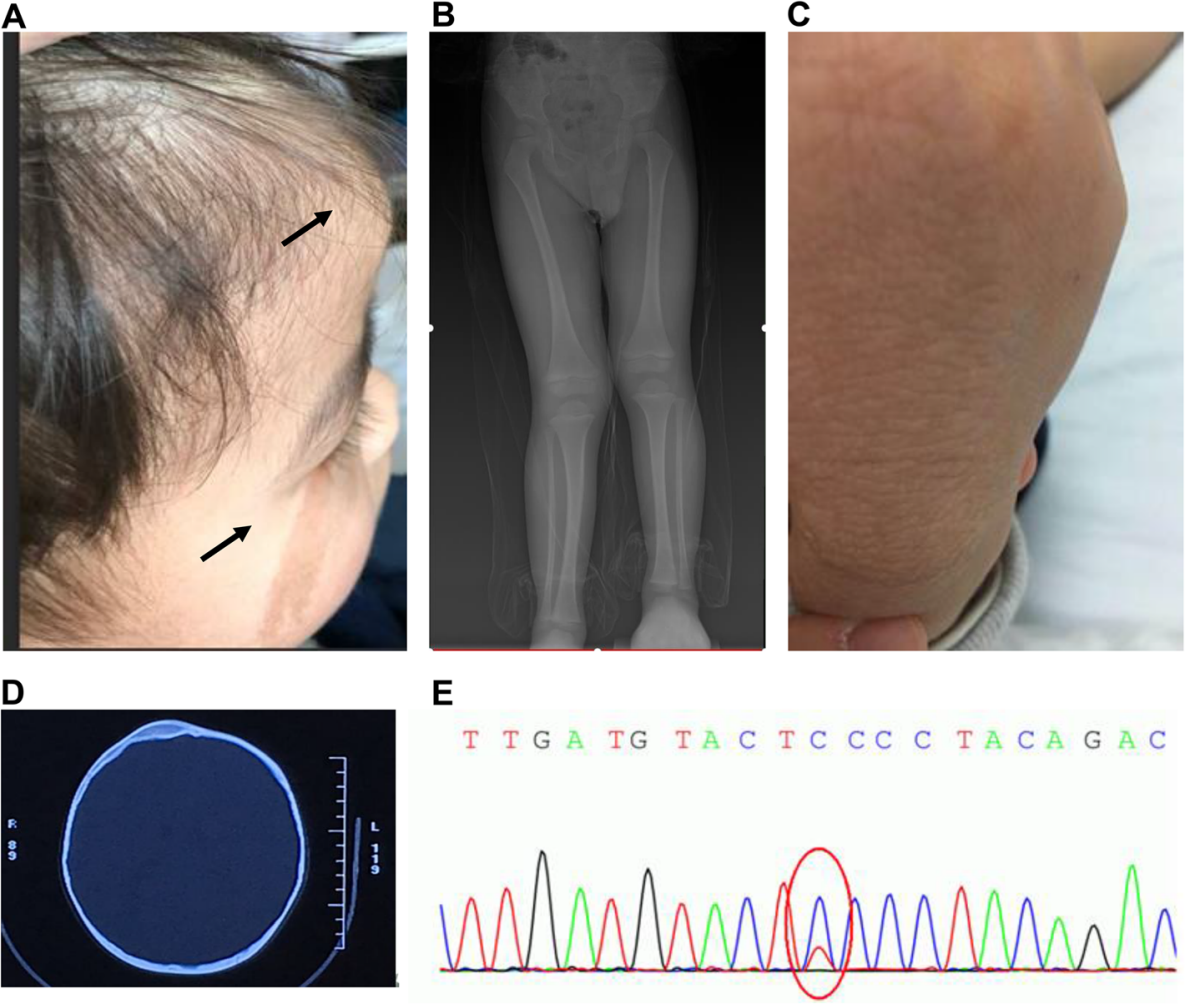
Representative images of the patient. a Milk coffee spots on the right facial skin (arrow) and right frontal bone bulging (arrow). b X-ray detection: the right lower extremity was longer than the left one. c Nodular thickening skin on right hand back. d Head CT detection: thickening of the local diploic space on the right side of the frontal bone, showing a glass-like density. e Disease gene screening of the lesion samples form the milk coffee spots, and nodular thickening skin at hands and feet. There was a heterozygous point mutation 49G > A (p.Glu17Lys) in the exon region of the AKT1 gene
